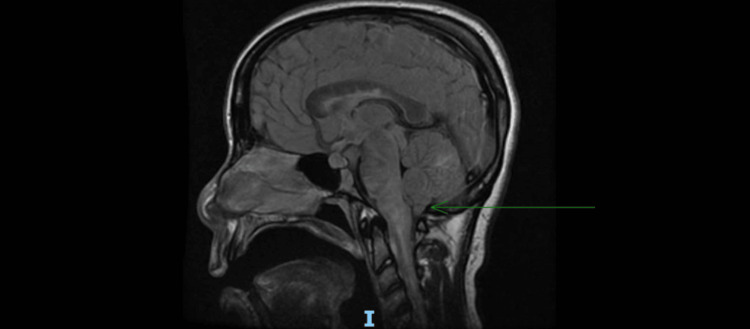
MRI of the brain T2 fluid-attenuated inversion recovery sequence (sagittal view) showing swelling of the brainstem and cerebellum with effacement of ventricles MRI: magnetic resonance imaging
